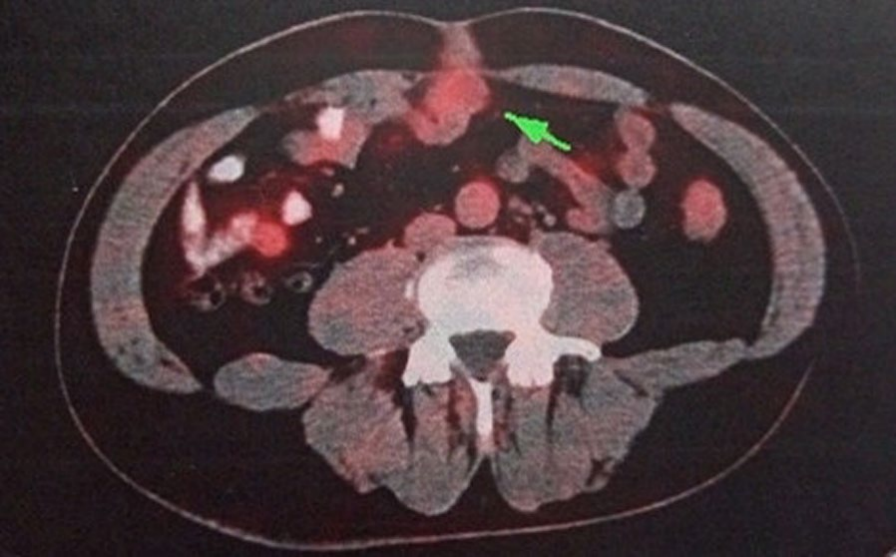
The PET–CT scan: umbilicus lump (*arrow*). An increased metabolic rate was revealed. Radioactive tracer: 18F-FDG
